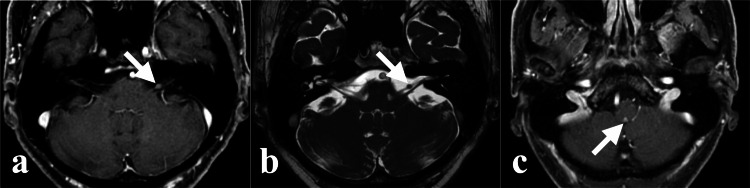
The left facial nerve and medullary lesions on axial MRI before CKR. (a) Enhanced lesion of the left facial nerve (indicated by the white arrow). (b) Nodular lesion of the left facial nerve on heavy T2WI (indicated by the white arrow). (c) Enhancement of the medullary lesion (indicated by the white arrow). CKR: CyberKnife radiotherapy, T2WI: T2 weighted image.
